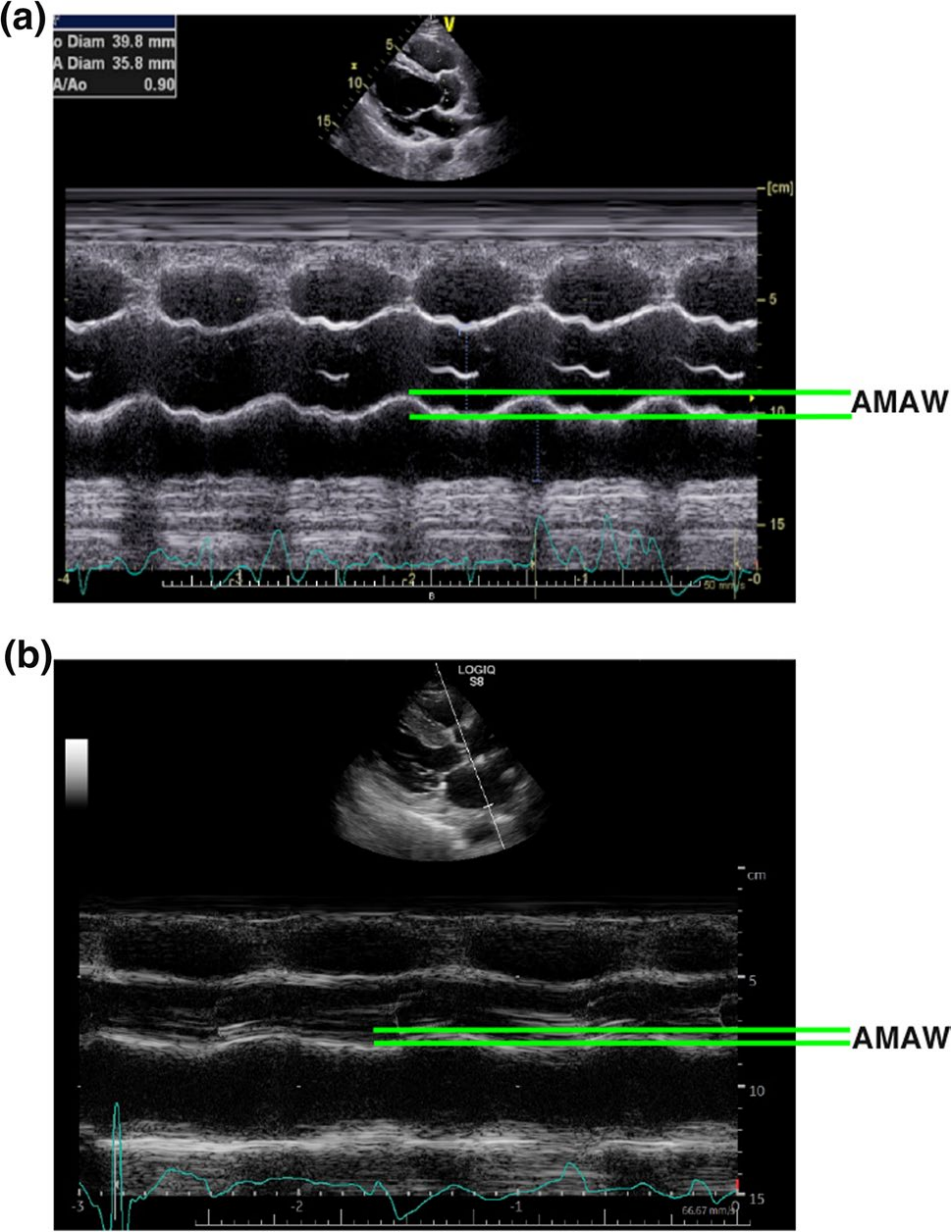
The amplitude of the posterior aortic wall was measured as the amplitude of motion of the posterior aortic wall at the aortic valve level on M-mode echocardiography in the parasternal long-axis view. a A male patient with cardiomyopathy in his 30 s. b A female patient with heart failure with preserved ejection fraction in her 90 s. AMAW varies across people. Abbreviation: *AMAW* amplitude of motion of the aortic wall
